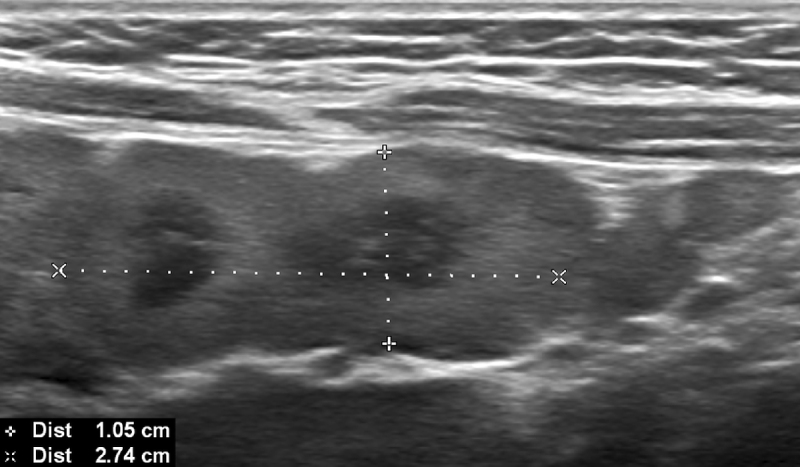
Greyscale ultrasound image demonstrating confluent lymph nodes with a supra-centimetric small axis.
